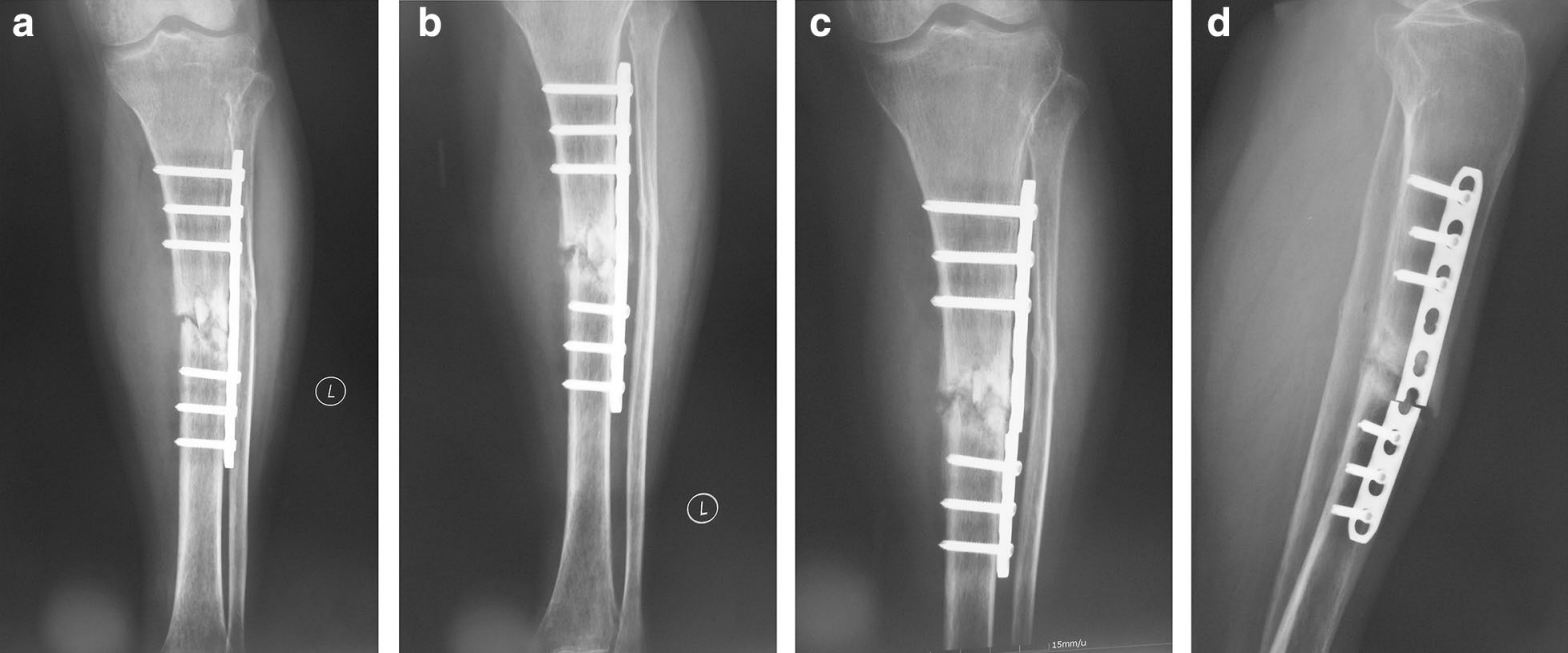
Anteroposterior radiographs of tibia and fibula fracture in 4 months (a), 6 months (b) and 7 months (c) after surgery. Lateral radiograph (d) taken 7 months after surgery showing the nickelclad breakage
